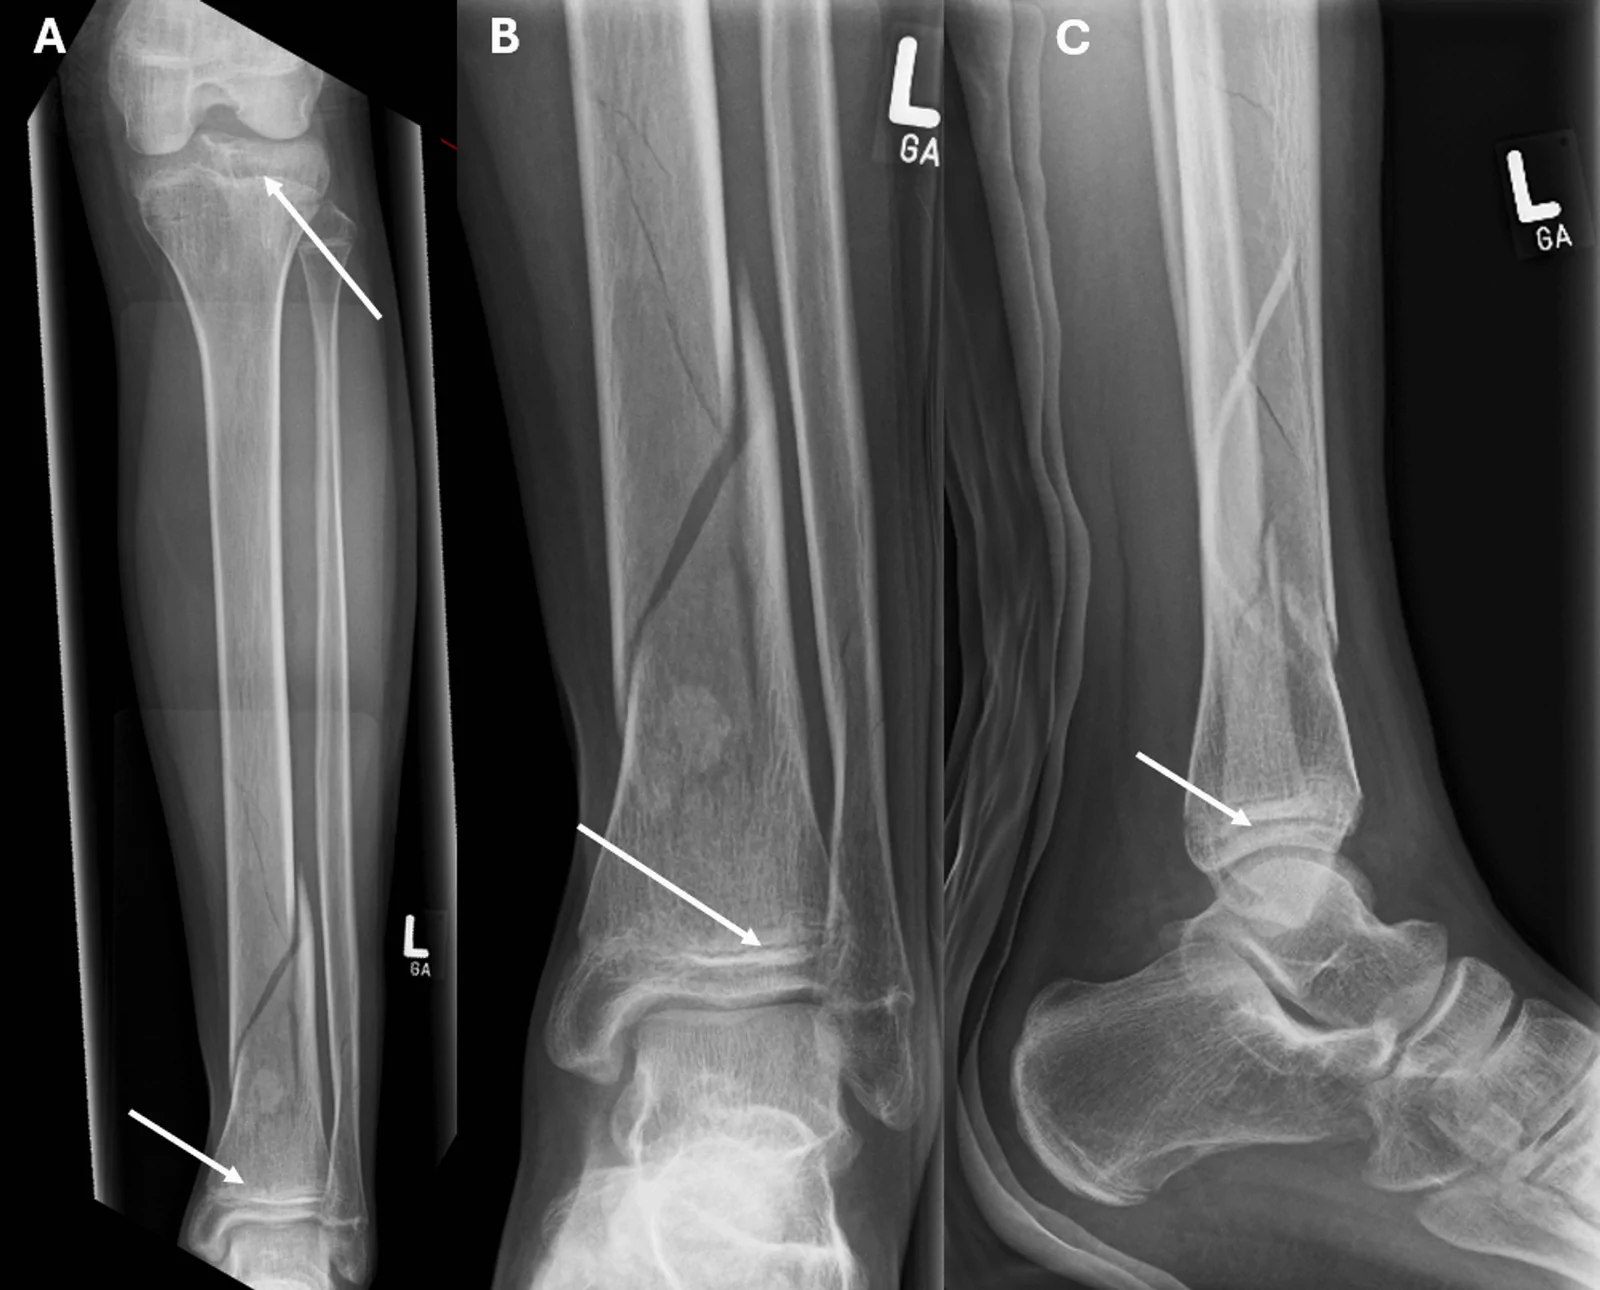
Preoperative radiographs of the left ankle showing a spiral fracture of the tibial shaft with persistently open growth plates (arrows indicate open physes at the proximal and distal tibia). (A) Anteroposterior long view; (B) Anteroposterior short view; (C) Lateral view
